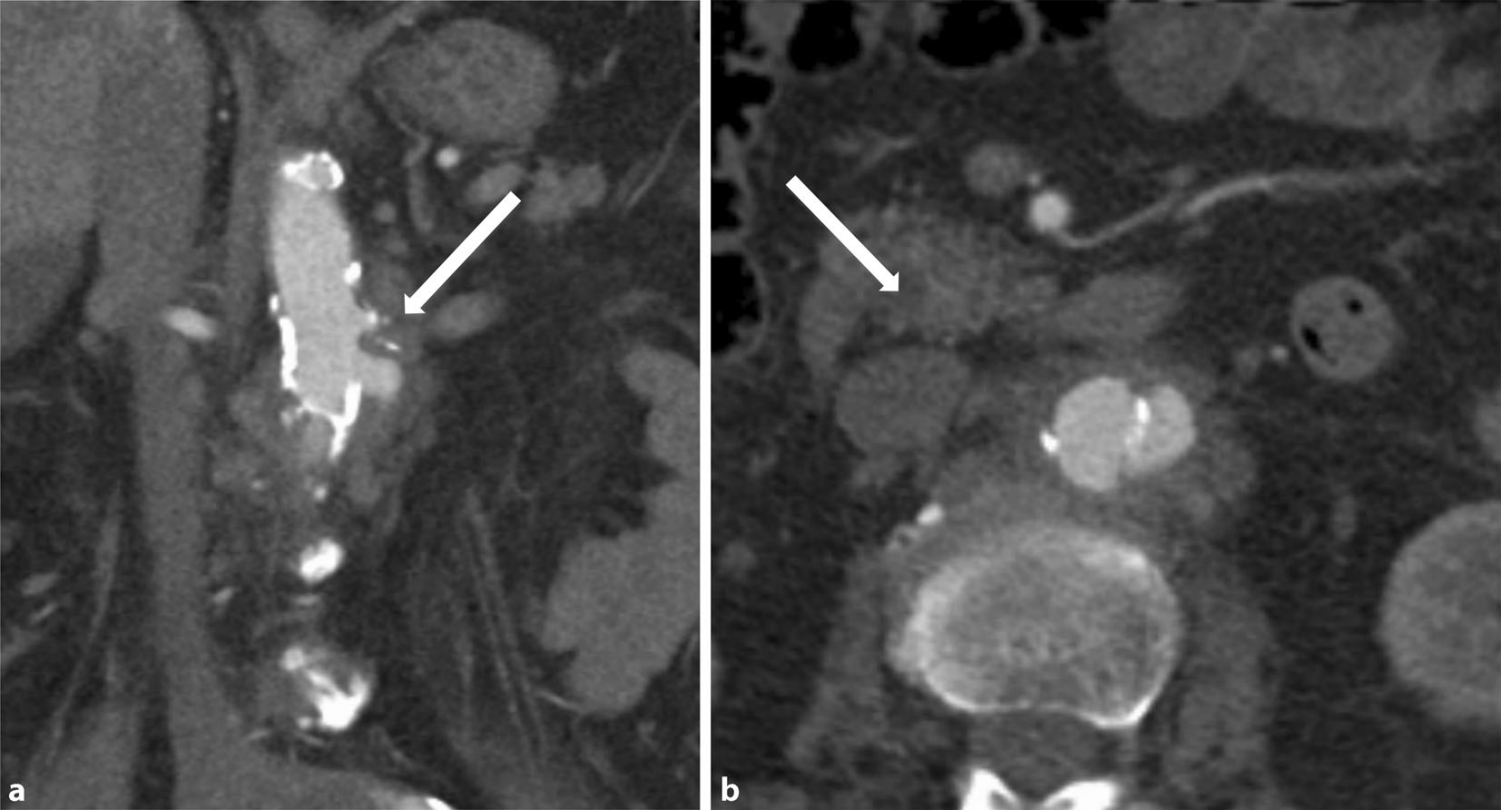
Abdominal computed tomography following intravenous contrast medium, arterial phase, coronal reconstruction. (a) Mycotic aneurysm of the aorta immediately below the branch of the left renal artery (*arrow*), (b) abdominal computed tomography following intravenous contrast medium, axial view, arterial phase. Visualization of the mycotic aneurysm with a breach in aortic wall calcification. Inflammatory wall thickening and patent inflammatory periaortic soft tissue border (*arrow*)
